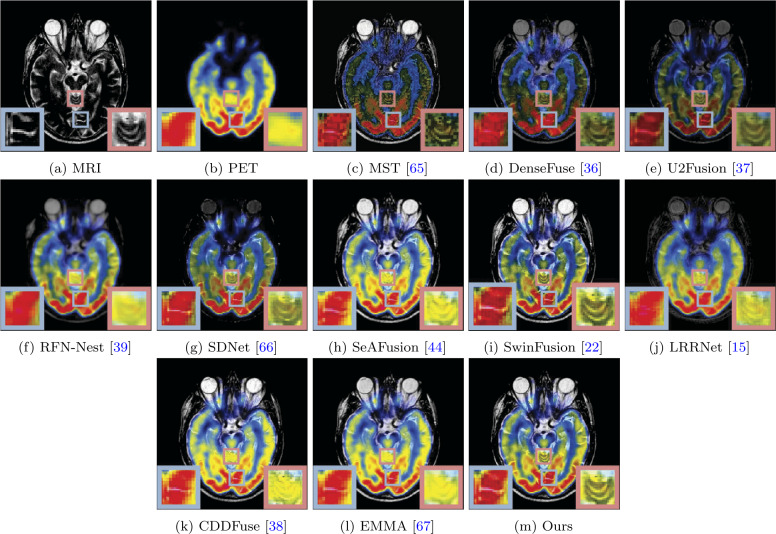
Visual comparison of our method with ten state-of-the-art methods on MRI and PET image fusion
